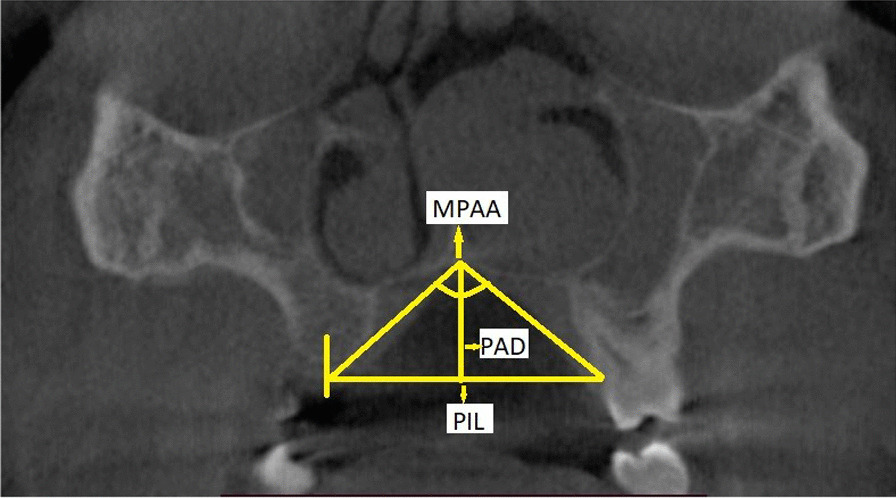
Coronal CBCT section showing landmarks for the palatal dimension measurements. The palatal interalveolar length (PIL) is the distance between the mid-centres of the cervical portion of the available tooth, from one side to the other. If there was no tooth, then the mid-centre of the alveolar bone near the crest was considered the reference point. Palatal arch depth (PAD) is the length of the line from "P" (junction of the nasal septum and hard palate) to the interalveolar line. The maxillopalatal arch angle (MPAA) is the angle that is formed by the lines from "P" to both points of the mid-centre of the available tooth or the midpoint maxillary alveolar bone for patients missing teeth
